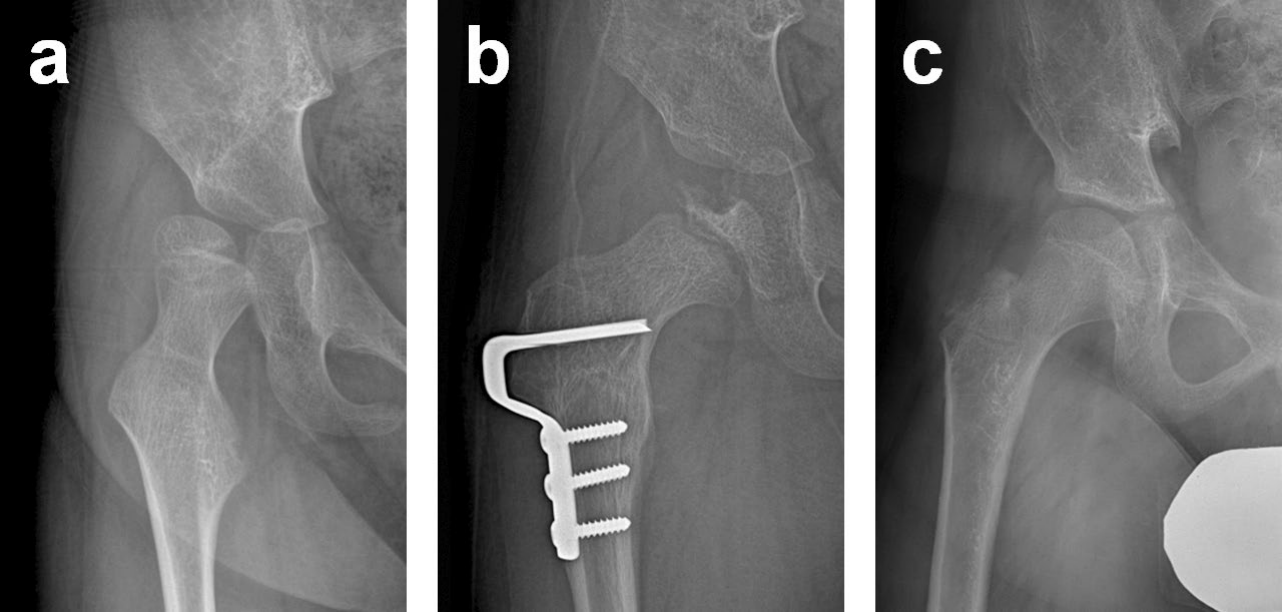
(a) Preoperative anteroposterior (AP) radiograph showing a subluxated hip in a 6-year-old boy. (b) AP radiograph showing Type 3 AVN developed after open reduction and varus derotational osteotomy of the proximal femur (VDRO). (c) At 6 years after surgery, the femoral head was noted to be remodelled without subluxation.
